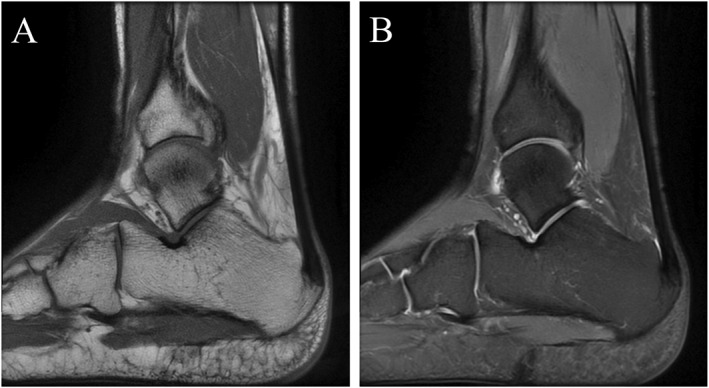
A 32‐year‐old male amateur marathon runner with a 5‐year running experience and a monthly running distance of 150 km; representative MRI of inserted Achilles tendinopathy. Sagittal T1WI (A) and fs‐PDWI (B) of the right ankle show calcaneal spur formation and linear hyperintense signal enhancement at the Achilles tendon insertion site.
